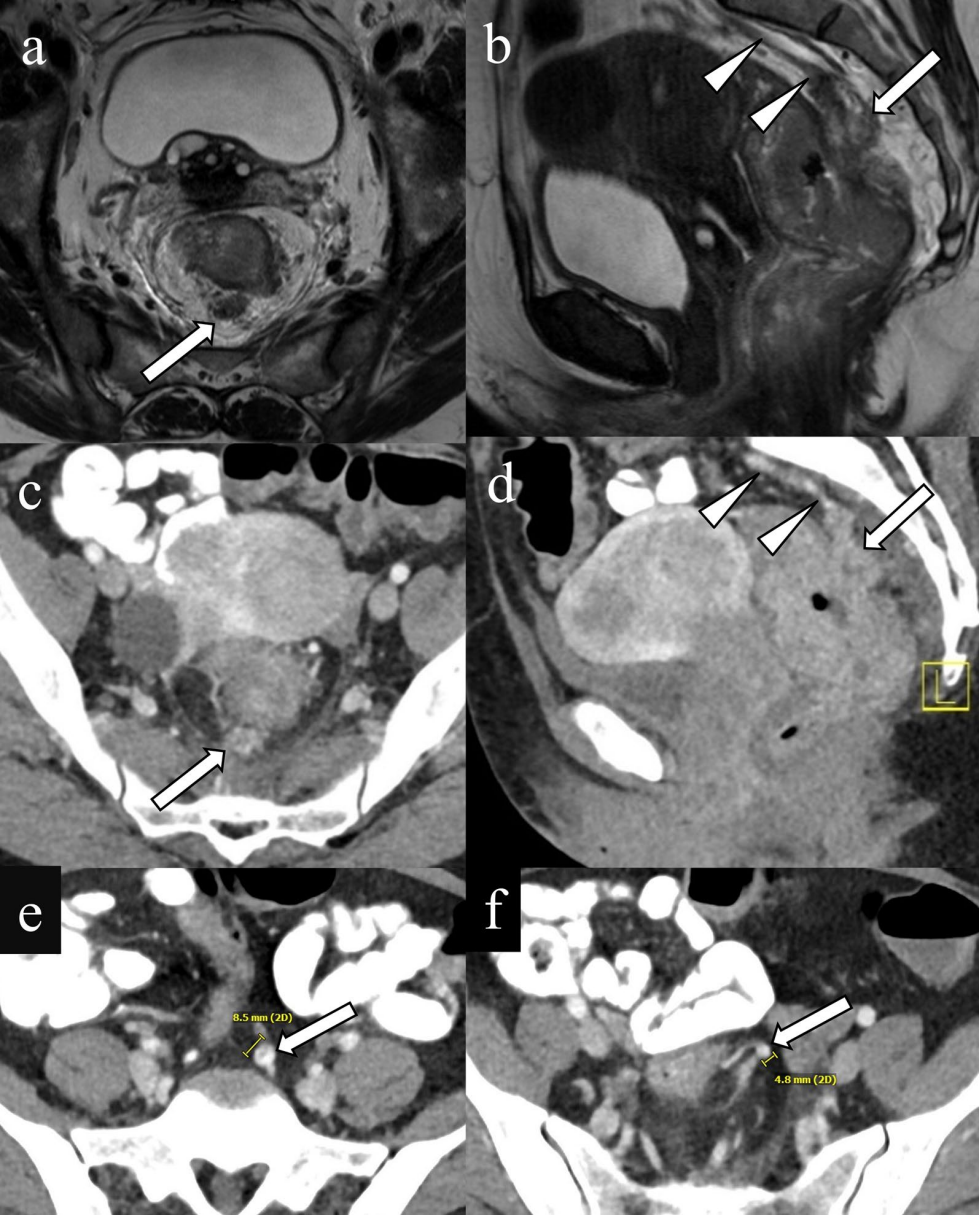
Extramural venous invasion on CT. A 47-year-old female with rectal adenocarcinoma. A nodule with an irregular margin containing intermediate signal intensity is observed in the mesorectum on axial T2WI (a arrow). Sagittal T2WI reveals a cord-like structure with tumor signal intensity in the superior rectal vein (b arrow) which drains to the inferior mesenteric veins (b arrowheads) Similarly, contrast-enhanced CT demonstrates an enhancing irregular nodule within the posterior mesorectum on the axial image (c arrow). On the sagittal image a cord-like nodular mass is contiguous with the dilated superior rectal vein (d arrow) and inferior mesenteric veins (d arrow heads) on the sagittal image. The diameters of the inferior mesenteric and superior rectal veins are 8.5 mm (e arrow) and 4.8 mm (f arrow), respectively, which is suggestive of the presence of EMVI
